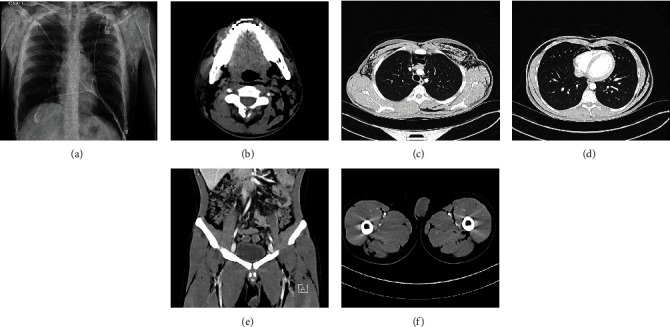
(a) Chest x-ray with pneumomediastinum with subcutaneous air in the chest wall and neck. (b) CT head with subcutaneous emphysema. (c, d) CT Chest with pneumomediastinum and extensive subcutaneous and soft tissue emphysema throughout the chest and back without signs of a pneumothorax. (e, f): CT Abdomen with pneumoperitoneum and extensive soft tissue emphysema dissecting into the pelvis and bilateral lower extremities beyond the inguinal ligament.
